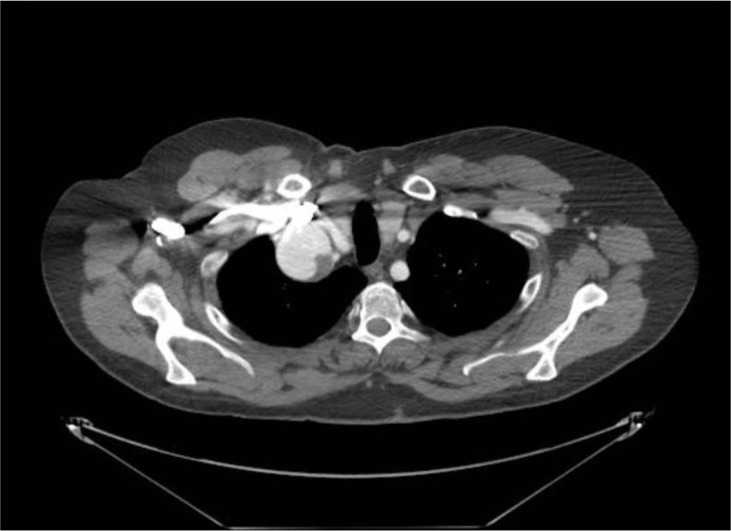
Axial computed tomography angiography demonstrating the size and location
